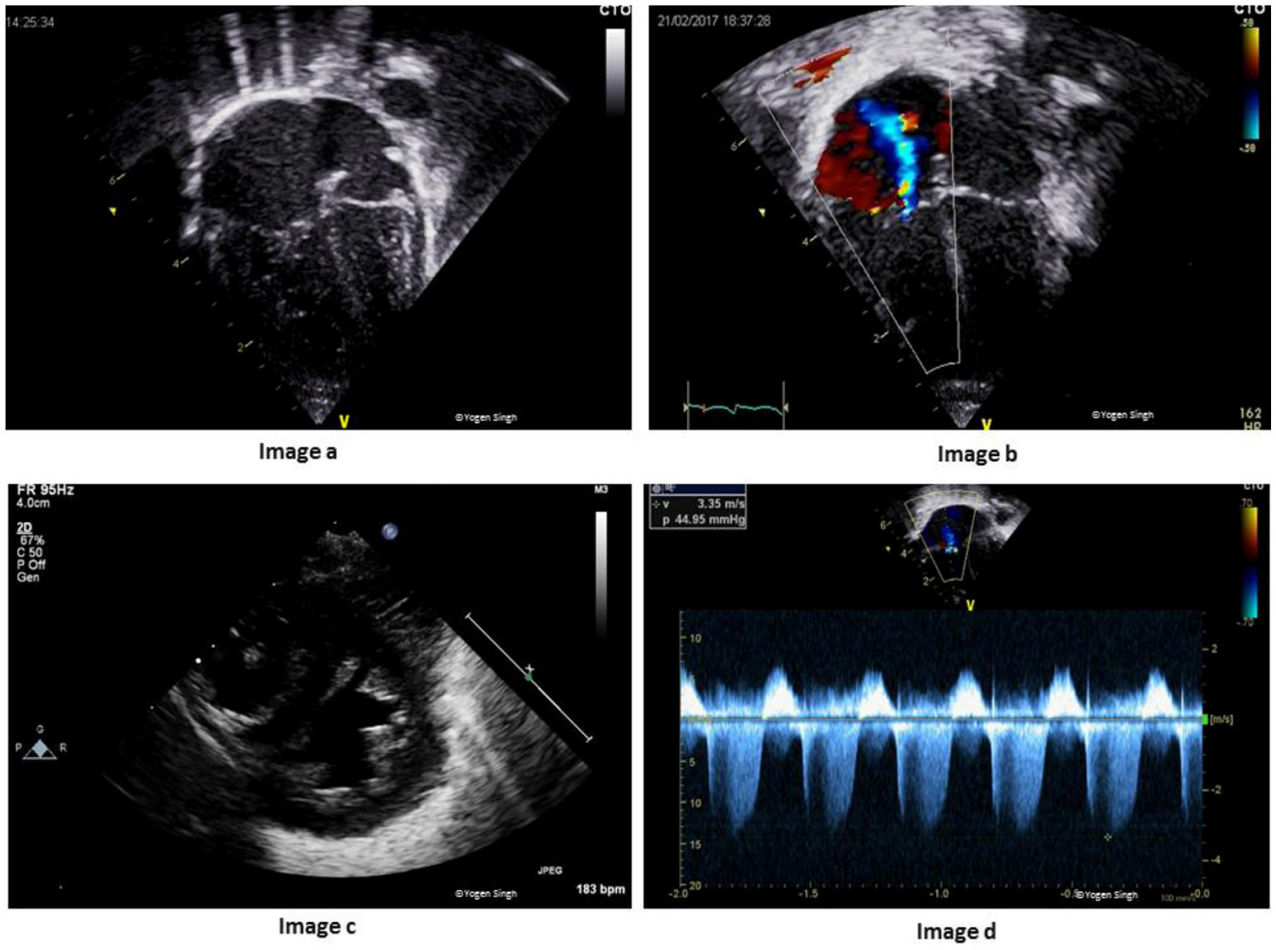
Echocardiographic assessment of pulmonary hypertension in adverse adaptation of transitional circulation. Image “a” shows right ventricular hypertrophy and mild dilatation in apical 4-chamber view; Image “b” shows tricuspid jet on color flow mapping; Image “c” shows hypertrophy of right ventricular and bowing of interventricular septum in parasternal short axis view; and Image “d” shows Doppler assessment of tricuspid regurgitation (TR) to assesses pressure gradient between right ventricle and right atrium. Pulmonary artery systolic pressure can be estimated by adding right atrial pressure to the pressure gradient measured by TR velocity.
